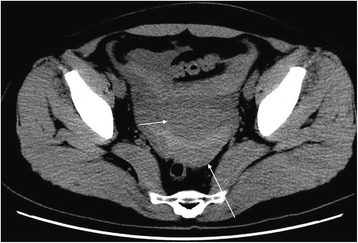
CT findings of REPWH. The patient presented with lower abdominal pain for a period of 2 h. This patient had a history amenorrhea for 2 months and irregular vaginal bleeding for 1 month; β-HCG test was positive; no signs of gestational sac were observed; left isthmus ectopic pregnancy was confirmed by surgery. The single arrow indicates the mixed-density pelvic effusion; the front shorter arrow indicates the location of the uterus, which does not exhibit any signs of cystic shadow
